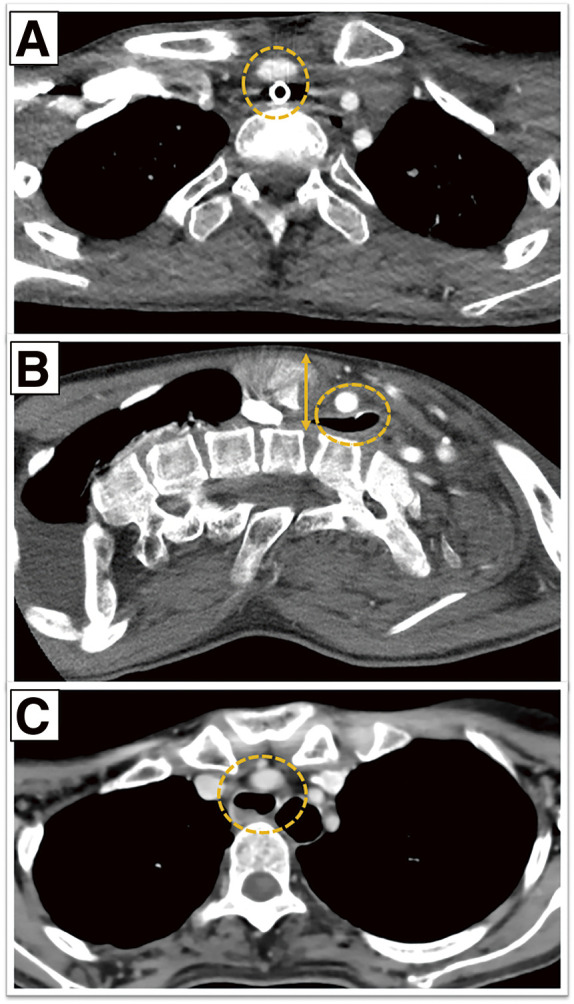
Preoperative contrast-enhanced computed tomography (CT) demonstrating impending tracheo-innominate artery fistula. (A) Axial view showing direct contact between the tracheostomy tube and the innominate artery (dashed circle). (B) Sagittal reconstruction demonstrating extremely narrow anteroposterior mediastinal space (18 mm) between the posterior surface of the sternum and the anterior surface of the cervical vertebra (arrow and dashed circle). (C) Axial CT image in another case showing close contact between the trachea and the innominate artery (dashed circle).
